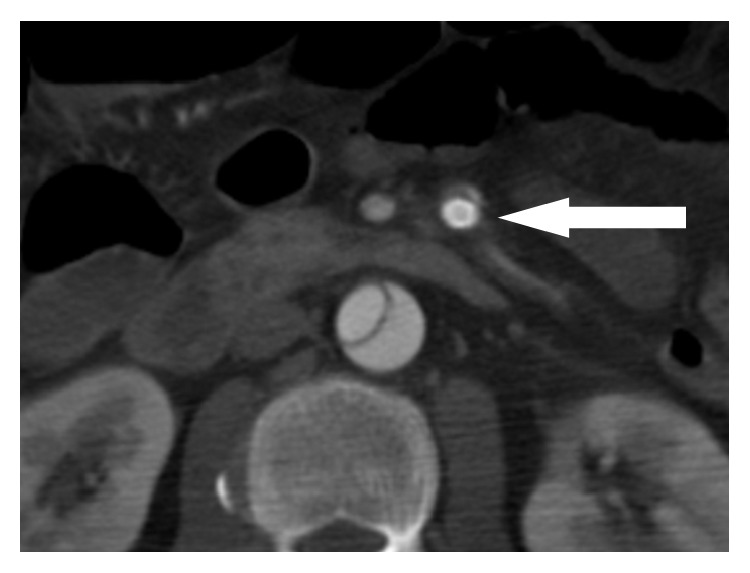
Contrast enhancement in the internal SMA stent was well maintained on contrast-enhanced CT at two weeks after the stenting.
